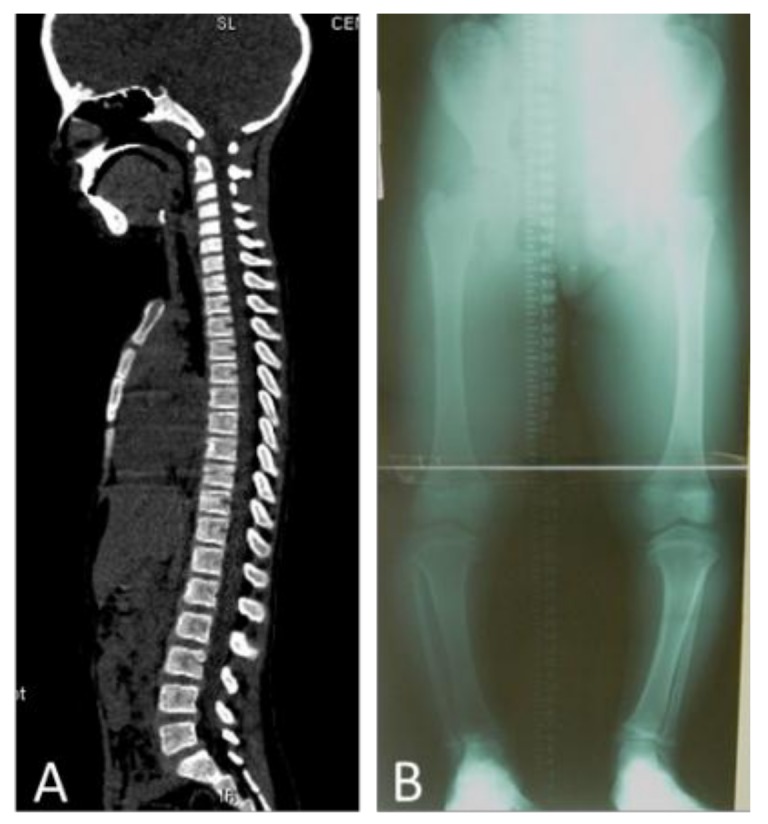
(A) 3DCT scan of the vertebral column (mid-sagittal) of a 17-year-old girl showed odontoid hypoplasia and square-shaped vertebral bodies with marked anterior end-plate dysplasia of the T9-11. (B) AP radiograph of the lower limbs (telemetric) in a 15-year-old girl showed leg shortening relative to the thigh, and metaphyseal widening of the inferior femora and the superior tibiae effectively causing the development of genu varum.
